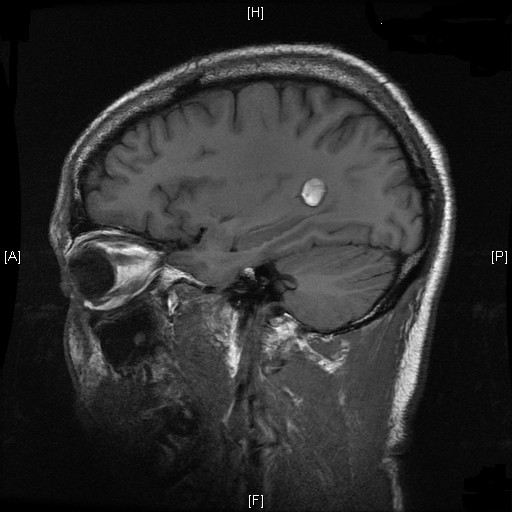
Magnetic resonance imaging of the patient, showing the area of haemorrhage.
